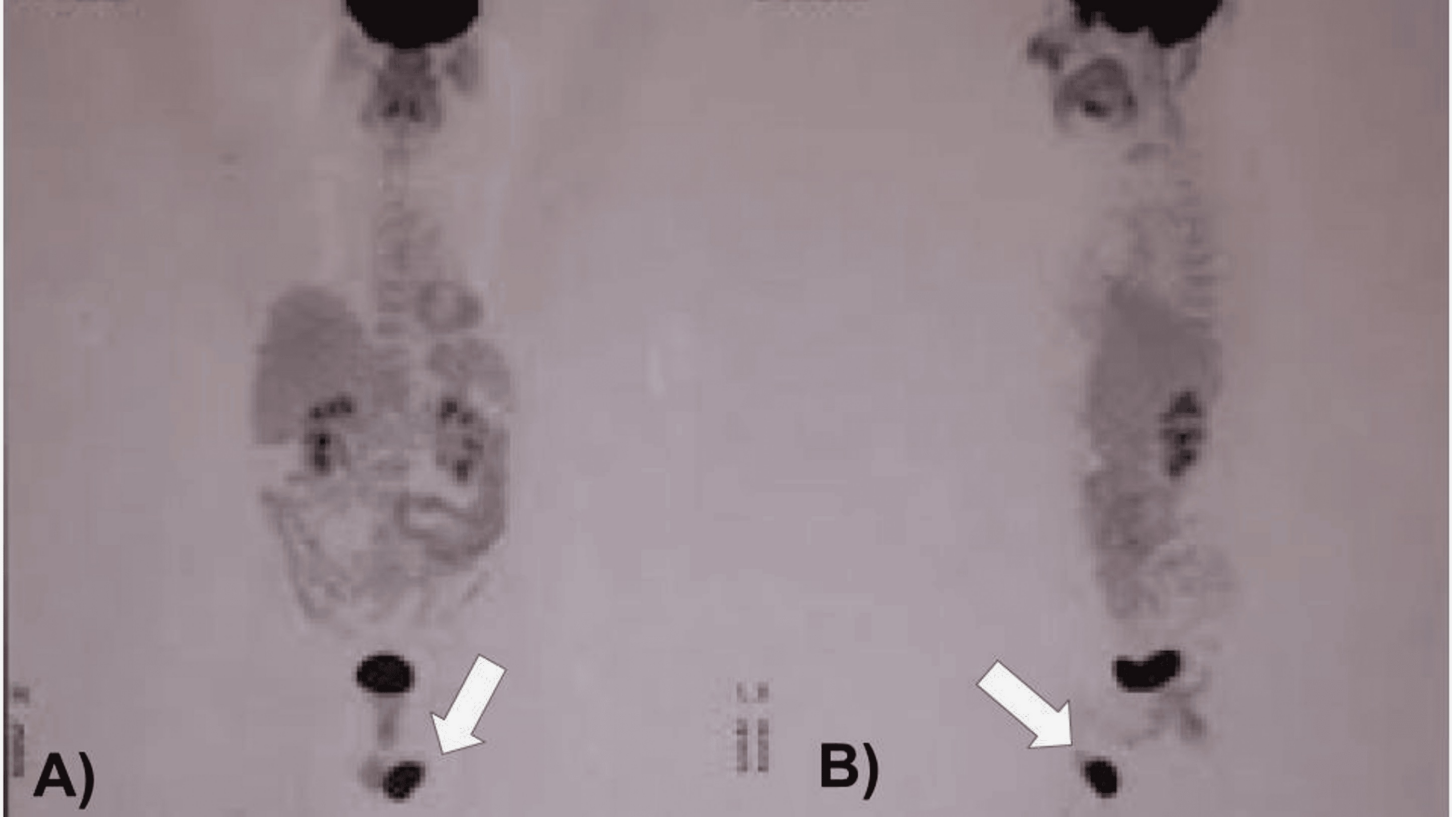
Body PET-CT with 18-fluorodeoxyglucose (FDG): (A) coronal and (B) sagittal image, with a white arrow in both images, show a solid lesion with lobulated edges in the left testicle, measuring 38 mm at the major axis, which is associated with focal hypermetabolism with a maximum standardized uptake (SUVmax) value of 32.6; no other alteration is observed.
